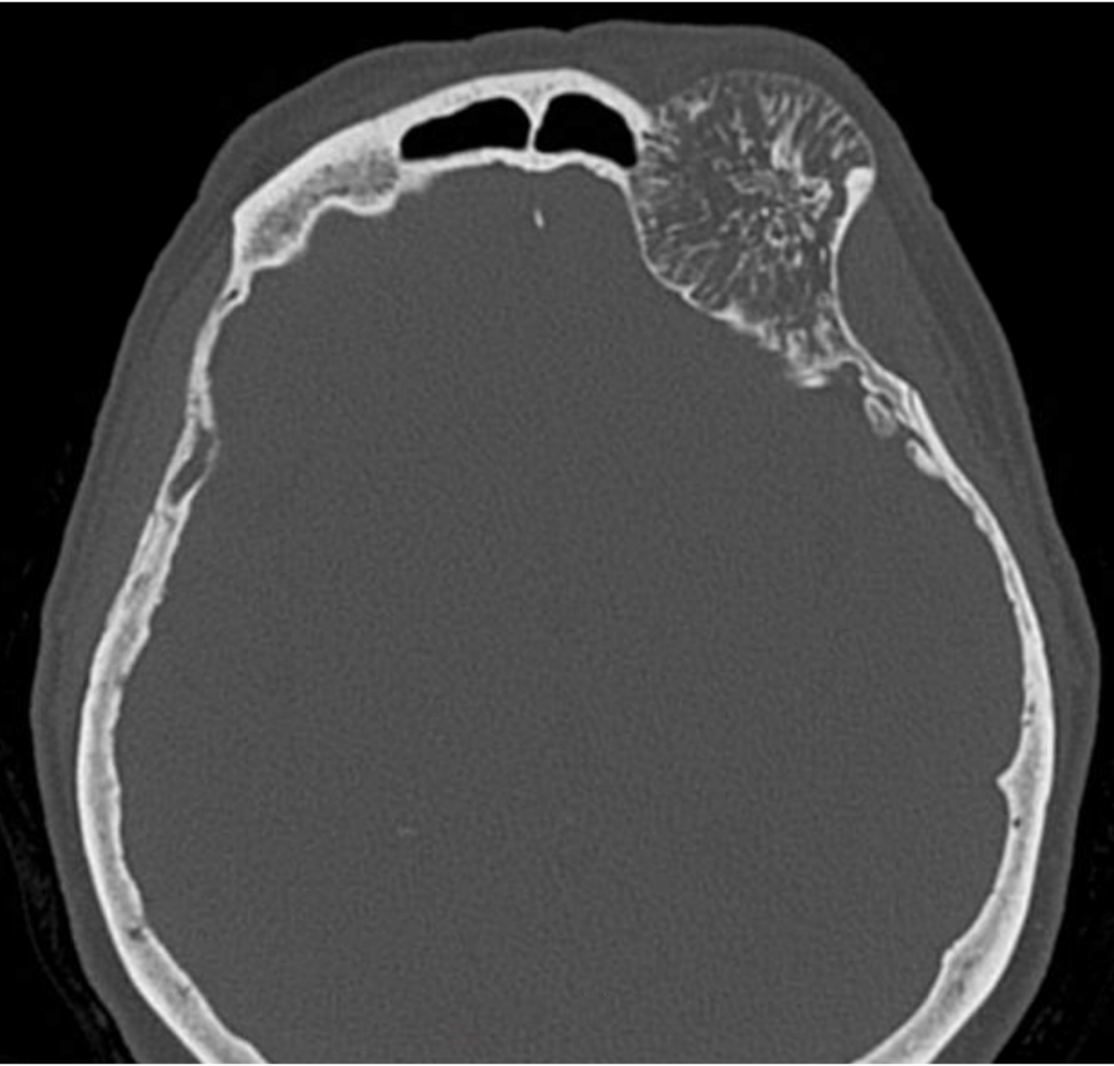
Preoperative axial computed tomography scan
